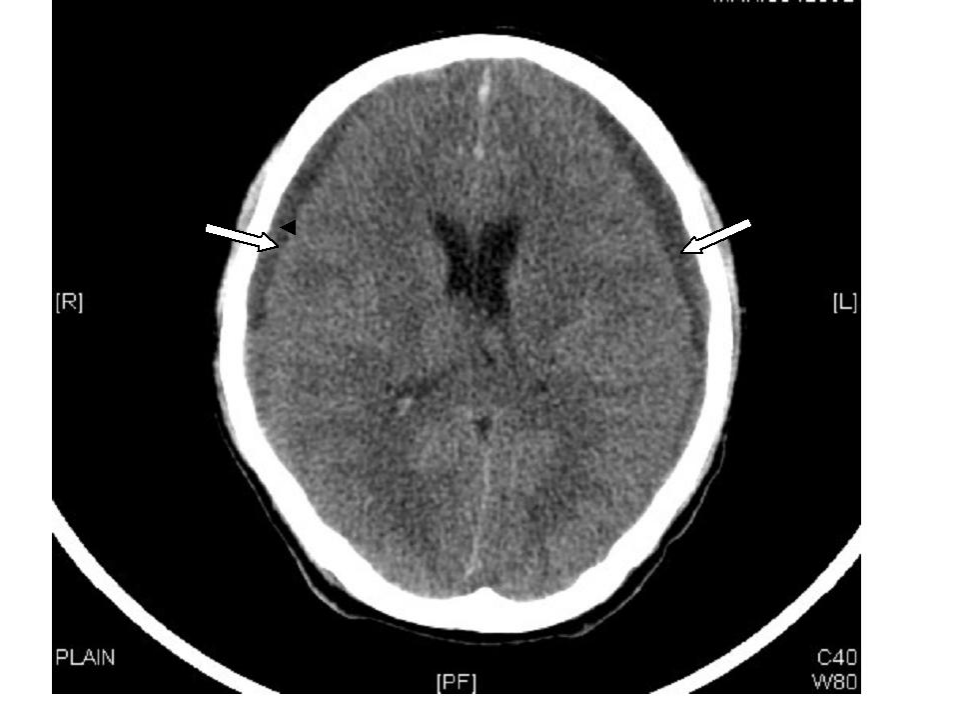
Plain computed tomography (CT) scan of the head showing bilateral subdural haematomas (marked by white arrows).
